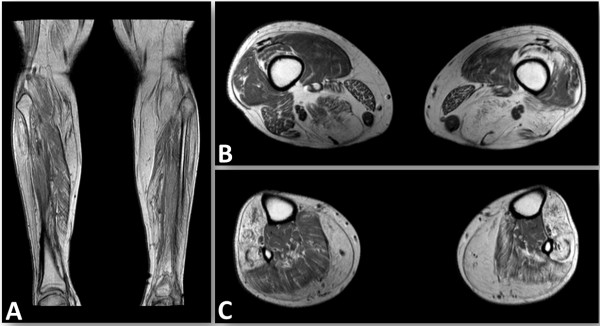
MR imaging. Whole-body MRI shows nearly complete fatty atrophy of the tibialis anterior muscles and the Mm. gastrocnemii, as well as moderate fatty replacement of muscle tissue in the soleus muscle (A, C). Atrophy of the dorsal upper leg muscles is pronounced in the M. semimembranosus and M. biceps femoris (B). Gluteal muscles revealed mild fatty degeneration. Additionally, fatty muscle degeneration of scapular fixators (Mm. serrati and Mm. latissimi dorsi) and axial back muscles was detected (data not shown). The pelvis and proximal upper legs were not examined as the patient had a hip implant.
